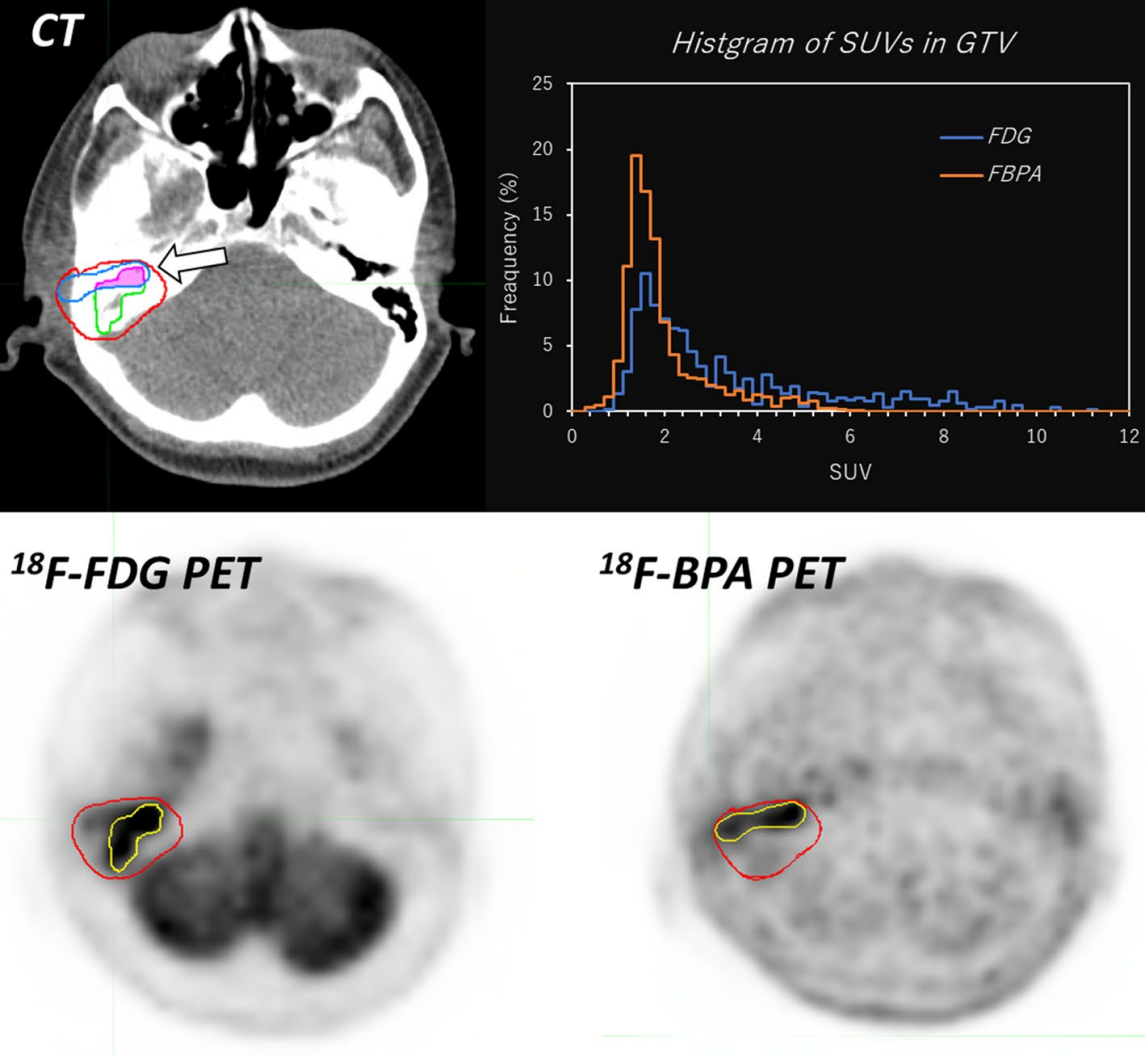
The example images of measure similarities (DSC, JC, and MDA) and histogram indices (skewness and kurtosis). The red and yellow lines on PET images (bottom images) show the contour of GTV and MTV40 on each PET image, respectively. The white arrow on the CT image indicates the overlap region between MTV40 on ^18^F-FDG and that on ^18^F-BPA PET. The overlap volume is used to calculate DSC and JC. The top right image shows histograms of SUVs derived from GTVs on both PET images for the calculation of skewness and kurtosis
